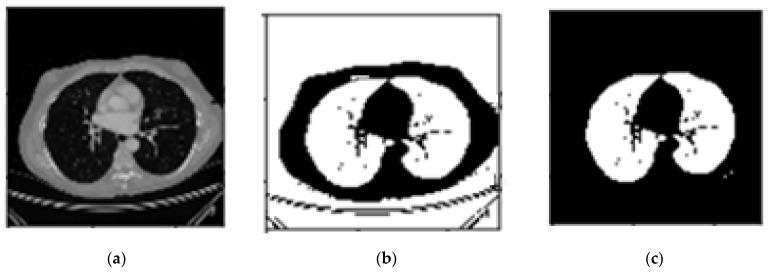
(a). Main CT image, (b). Binary image, (c). Image after eliminating border blobs.
